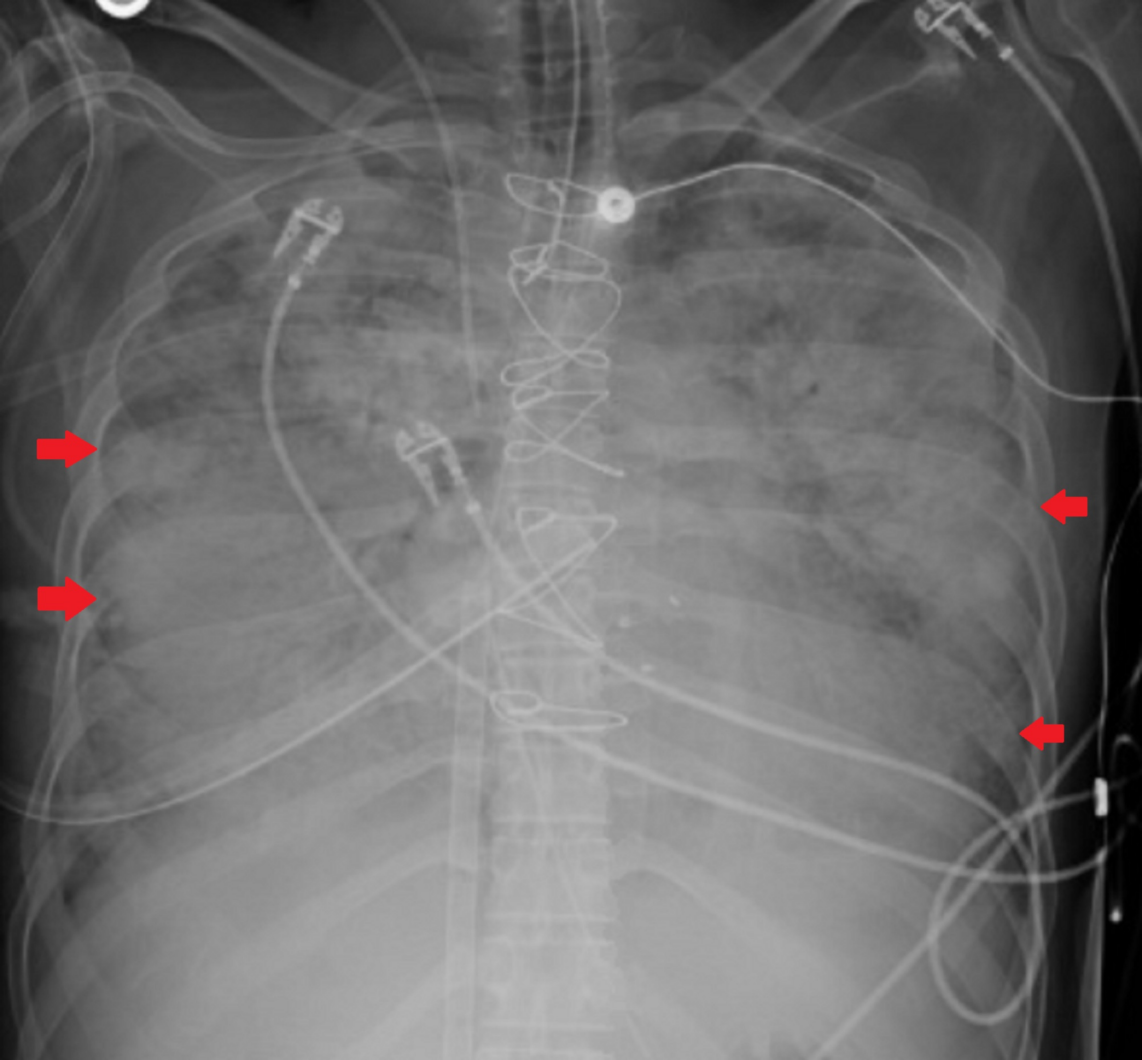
Chest x-ray with worsening bilateral pulmonary infiltrates
